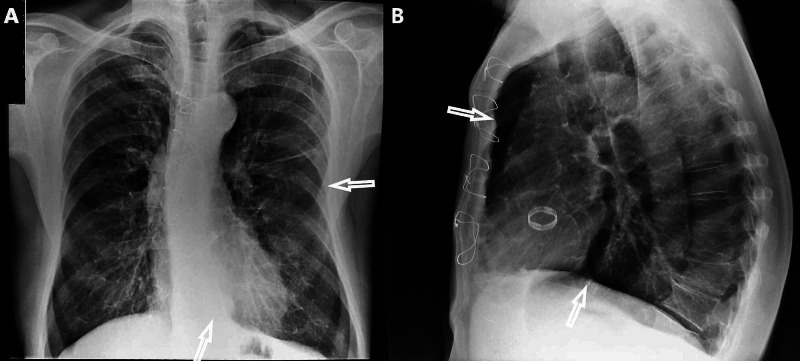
Chest X-ray showing emphysematous changes A. Arrows show horizontal ribs and small size of the heart. B. Arrows show increased retrosternal space and flattened hemidiaphragms.
